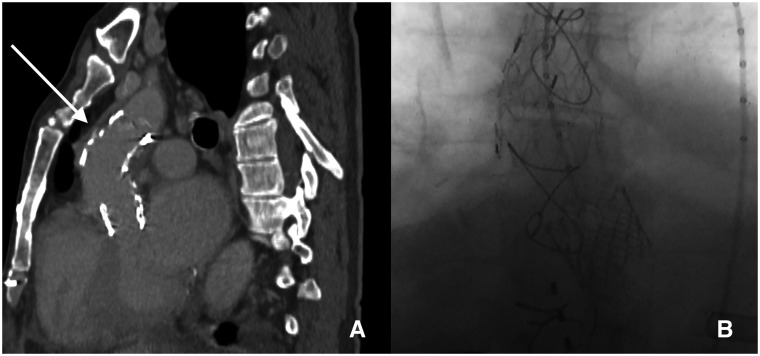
Postoperative imaging. (A) Computed tomography angiography scan revealing correct stent graft position and pseudoaneurysm exclusion (white arrow). (B) Final result: X-ray view.
